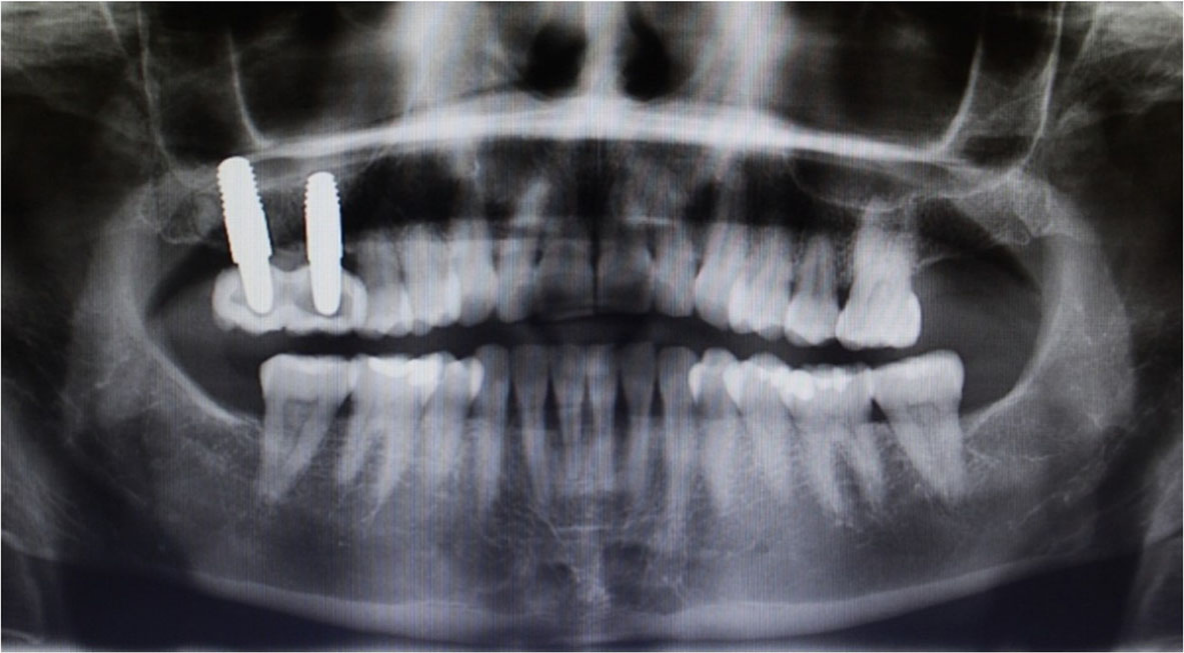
Follow-up after 1 year, no radiographic sign was appreciating and the osseointegration was satisfactory
